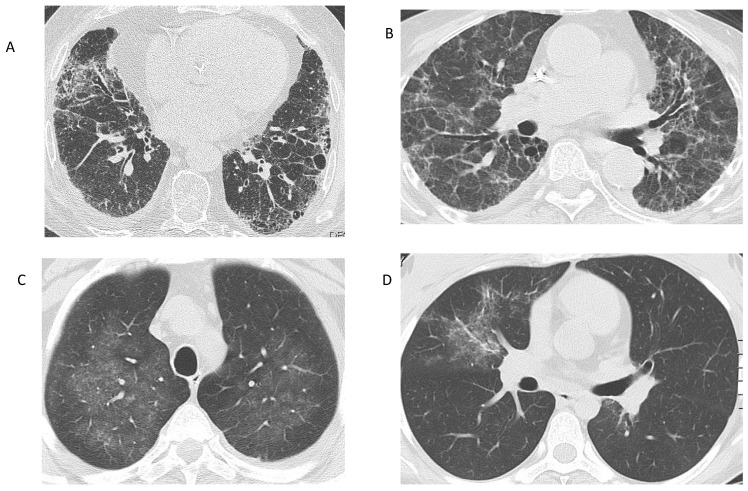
Radiographic findings in AAV. (A) Interstitial lung disease in a patient with MPA. Reticular opacities at the basal and peripheral lung segments, corresponding to a UIP pattern. (B) Interstitial lung disease in a patient with MPA, who presented with acute renal failure. Reticular changes and ground-glass opacities, suggestive of an NSIP pattern. (C) Ground-glass opacities in a patient with GPA: Bronchoalveolar lavage revealed alveolar hemorrhage. (D) Ground-glass opacity in the right upper lobe of a patient with EGPA, representing eosinophilic infiltration of the lung. (Images from the personal archive of Prof. Steiropoulos).
